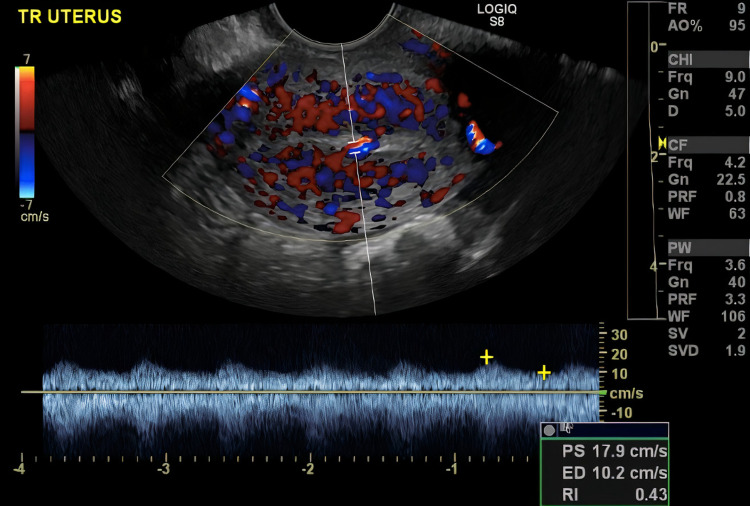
Transvaginal ultrasound with Doppler demonstrating uterine arteriovenous malformation.
